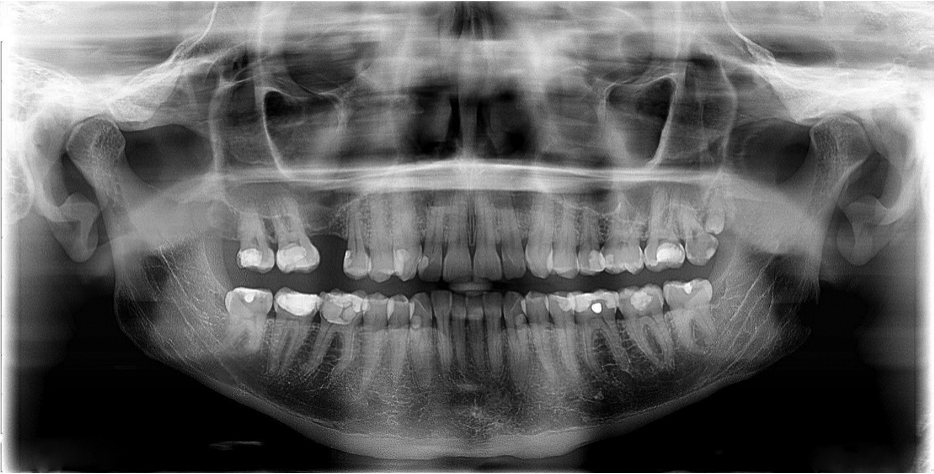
Case 2 - Bilateral bifid mandibular canal in Panoramic radiograph.
